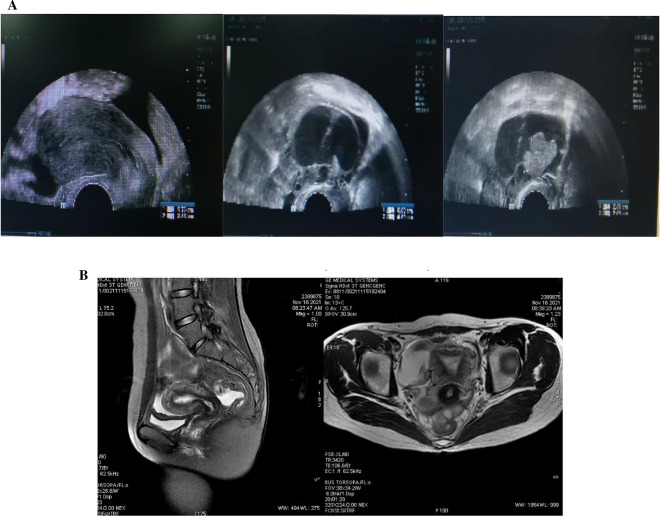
MRI Pelvis (Second Visit).
